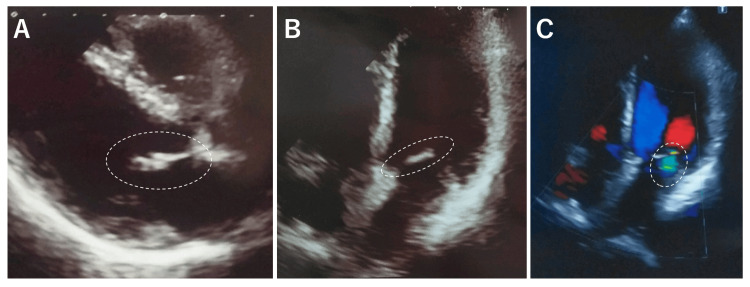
Echocardiogram showing possible anterior mitral leaflet calcification with mild mitral regurgitation at 4 weeks after the first hospital visit (A) Long-axis view suggested the presence of anterior mitral leaflet calcification (white ellipse). (B) Four-chamber view. (C) Four-chamber view in color Doppler echocardiography showing the presence of mild mitral regurgitation (white ellipse).
